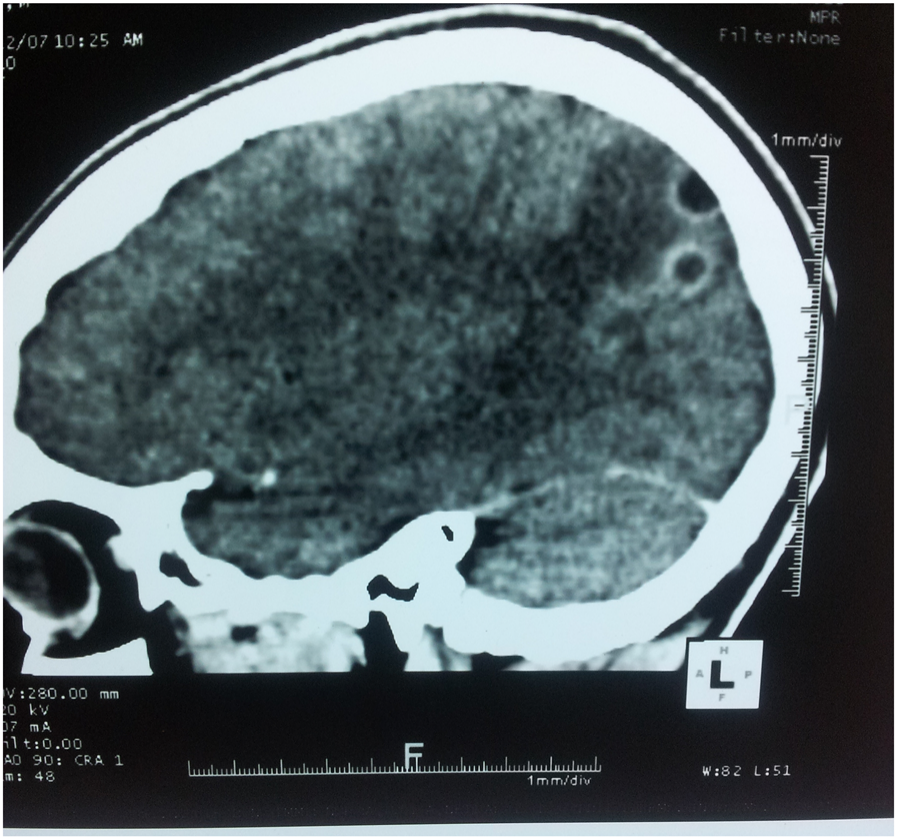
Contrast-enhanced CT showing two occipital cystic images with ring enhancement and perilesional edema.
